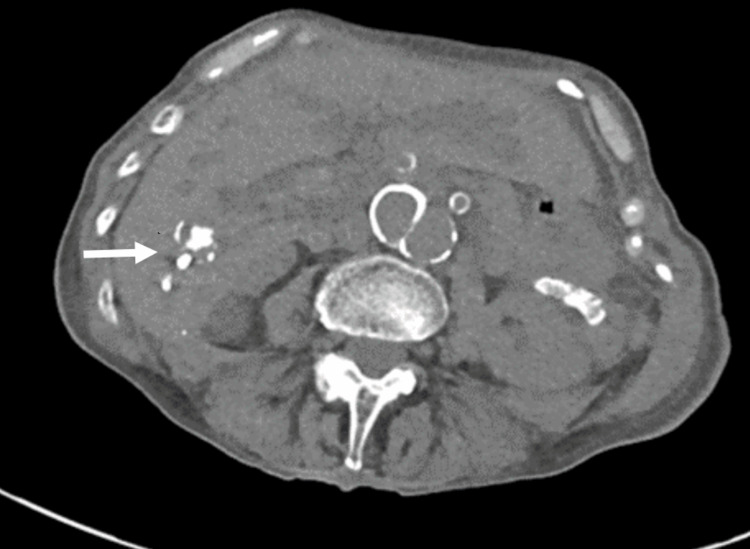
CT AP showing bleeding from the aneurysm causing haemobilia
